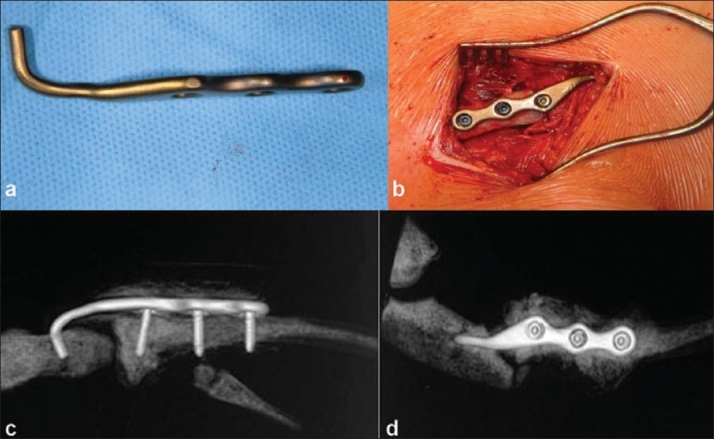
(a) Hook plate (Litos, Hamburg, Germany) after modification to suit the anatomical situation at the sternoclavicular joint; (b) intraoperative photograph showing repositioning of hook into the burred hole in sternum and the plate fixation with three cortical screws. (c) and (d) The postoperative CT showing a perfect reduction of the medial clavicle and the sternoclavicular joint
